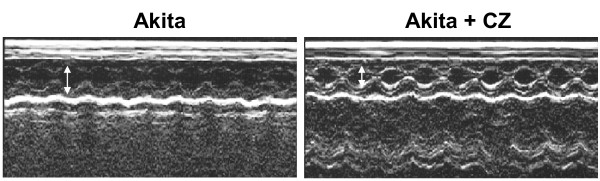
Diastolic diameter of Ins2+/- with and without treatment with ciglitazone. The echocardiogram shows amelioration of diastolic diameter (double headed arrow) of Ins2+/- after treatment with ciglitazone.
